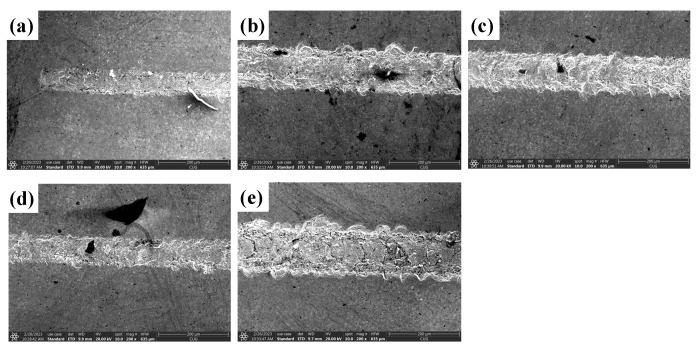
Surface morphology of ultrasound (ultrasound amplitude 5.07 μm) scratches at different positions. (a) show the surface morphology at the start of the scratch; (b–d) show the sur-face morphology at the middle of the scratch; (e) show the sur-face morphology at the end of the scratch.
